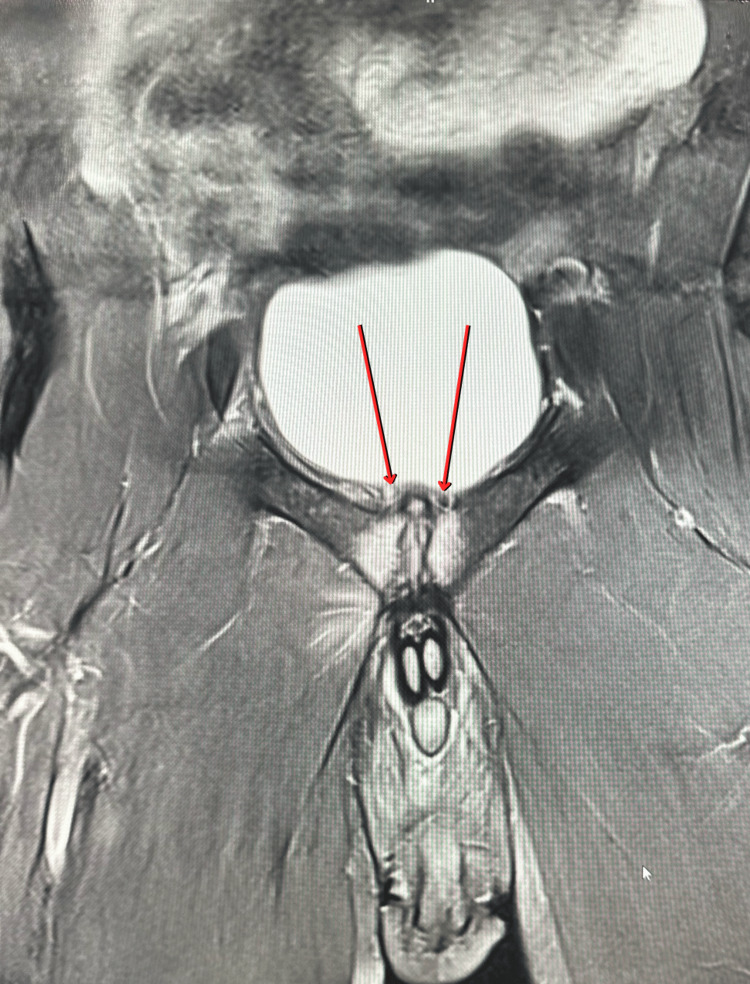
Coronal pelvic MRI (T2 fat-saturated/STIR sequences), demonstrating pubic symphysis edema (see red arrows) MRI: magnetic resonance imaging; STIR: short-tau inversion recovery
